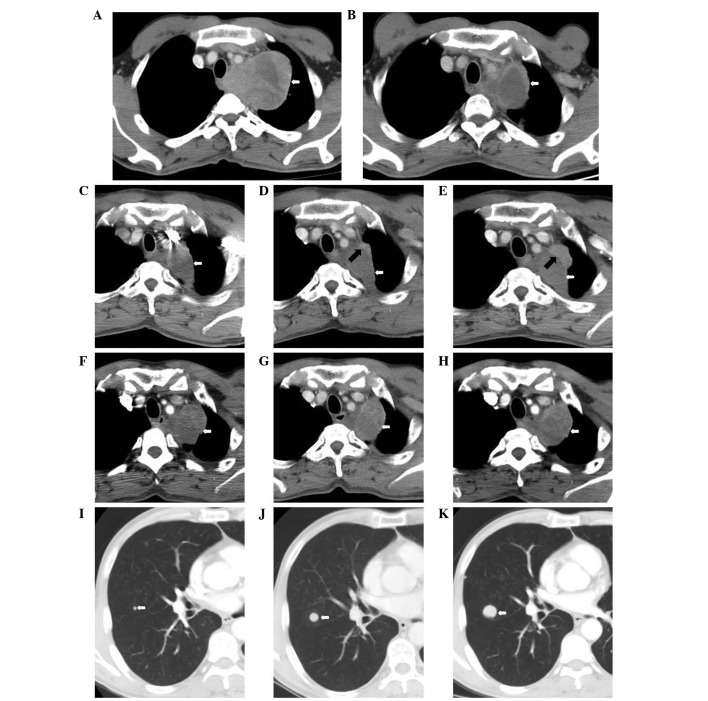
Chest computed tomography (CT) during the clinical course. (A) Pre-treatment on July 29, 2011 of the left lung apex to the middle and posterior mediastinum. The area of the largest cross-section is 7.9×10.0 cm (white arrow). (B) Post-concurrent chemoradiation on September 16, 2011. CT of the soft-tissue mass shows a maximal cross-sectional area of 5.3×4.6 cm (white arrow). (C) November 22, 2011, the left lung apex to the middle and posterior mediastinum following four cycles of paclitaxel + cisplatin chemotherapy and concurrent chemoradiation. The area of the largest cross-section is 3.3×4.2 cm (white arrow). (D) March 7, 2012, pulmonary primary lesions of the anterior and lateral to the adjacent irregular nodules following five cycles of docetaxel + nedaplatin chemotherapy. The area of the largest cross-section is 0.7×2.1 cm (black arrow). (E) May 14, 2012, pulmonary primary lesions of the anterior and lateral to the adjacent irregular nodules. The area of the largest cross-section is 1.6×3.1 cm (black arrow). (F) July 26, 2012 and (G) September 13, 2012, the left lung lump of the largest cross-section is 4.9×4.8 cm (white arrow). (H) October 25, 2012, the left lung lump of the largest cross-section is 5.0×4.0 cm (white arrow). (I) July 26, 2012, the nodes in the image demonstrate nodules of metastatic carcinoma and the largest is ~0.4 cm. (J) September 13, 2012 and (K) October 25, 2012, the nodules of metastasis increased and enlarged. The largest were ~1.2 and ~2.1 cm, respectively.
